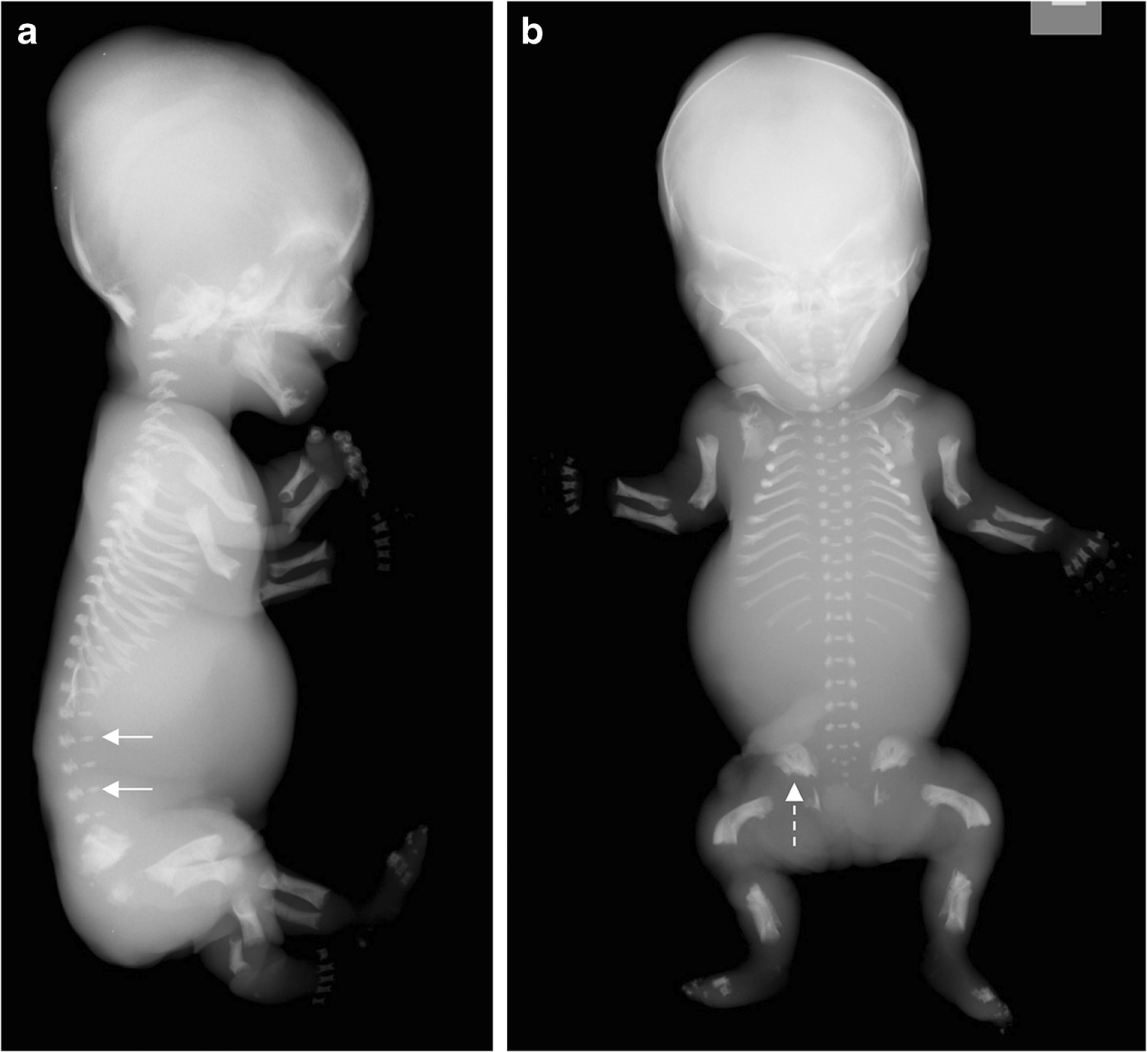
Radiographic features of a 21 weeks’ gestation male fetus with thanatophoric dysplasia type 1*.*a Lateral radiograph shows significant platyspondyly (*arrows*), with bowing of the humeri, femora and short ribs and a small thorax. b An anteroposterior radiograph shows horizontal acetabula (*dashed arrow*)
